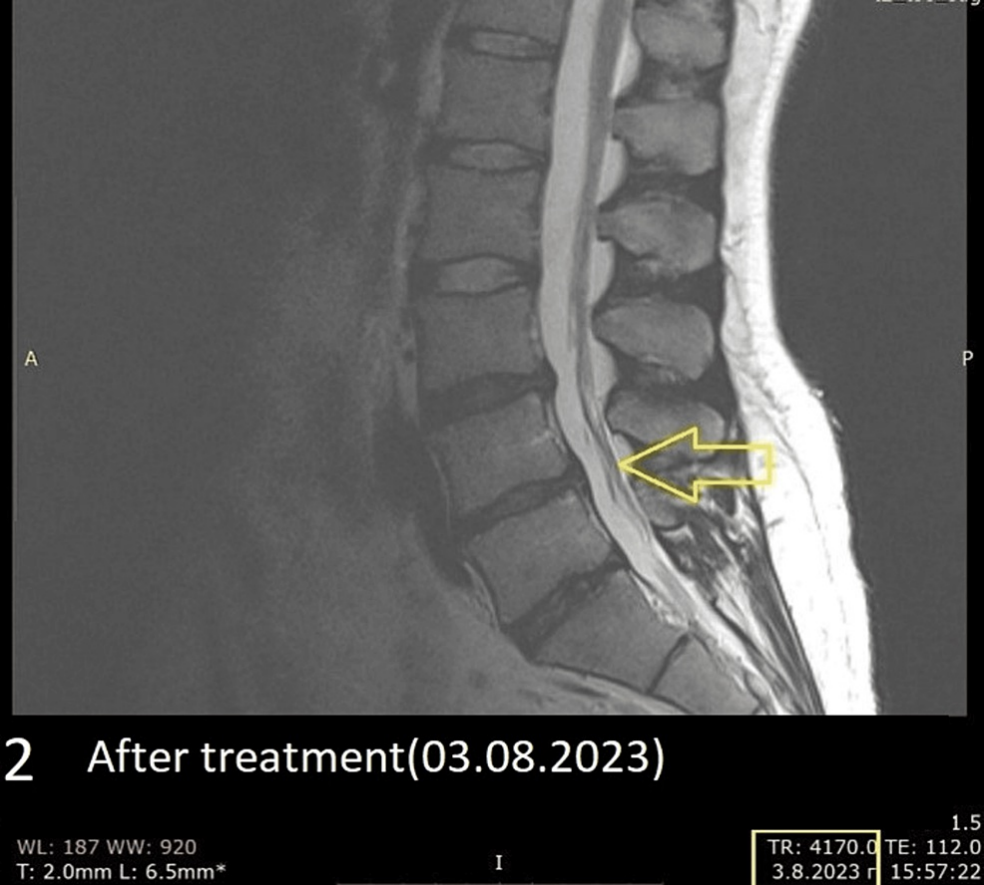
An MRI (sagittal plane) was performed seven months later without any surgical intervention; with conservative treatment, the herniation was gone (Date: 03.08.2023).
